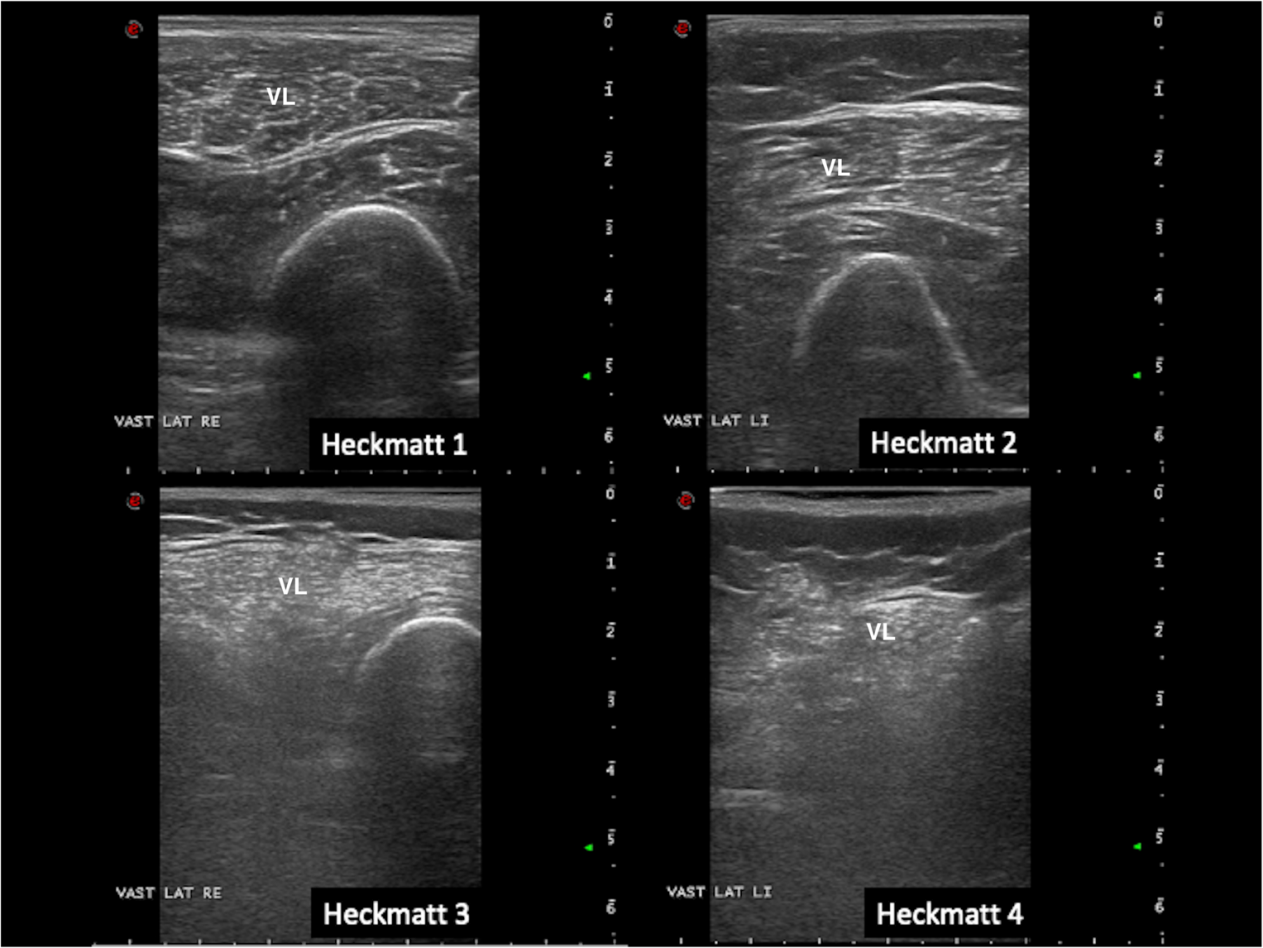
Heckmatt scoring using vastus lateralis muscle (disease examples from established myositis patients); grade 1 is normal; grade 2 shows a slight increase in echogenicity without architecture loss or attenuation. Grade 3 shows clearly increased muscle echogenicity, loss of muscle architecture, and some attenuation causing less visibility of deeper structures. Grade 4 shows a completely white muscle with loss of recognizable features and strong attenuation of the ultrasound signal so no deep structures can be discerned beyond the superficial layer of muscle. VL, vastus lateralis
